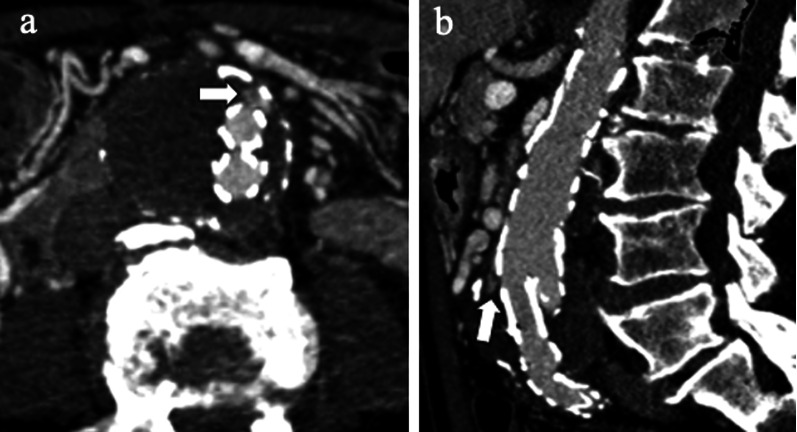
82-year-old man with *type IIIa* endoleak. a Axial and (b) sagittal CT angiography images show contrast agent outside stent-graft (arrows) close to the junction of the main body with the iliac limb
